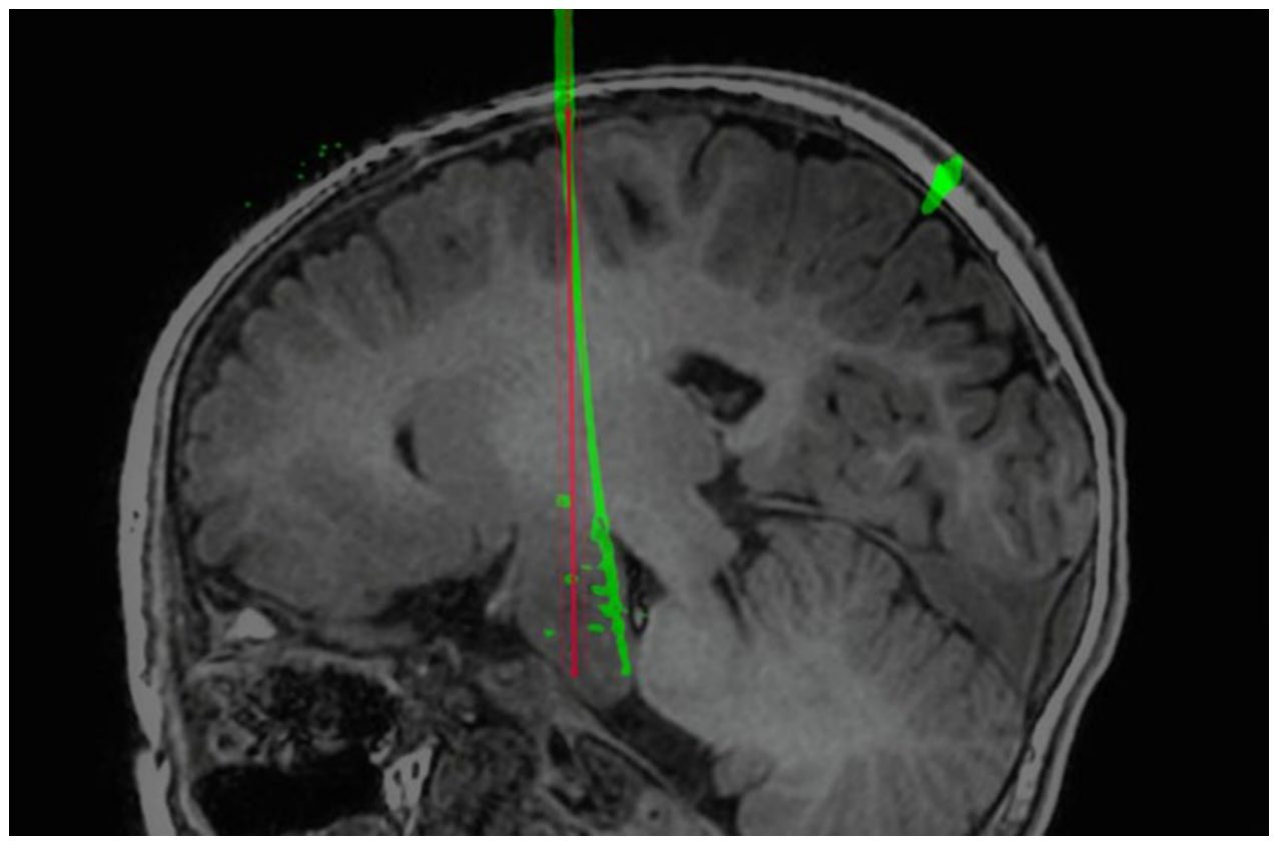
Example of self-bending of an intracranial electrode. The electrode spontaneously bent in the brain. The red line is the planned electrode track, and the green line is the actual electrode track in postoperative CT after reconstruction.
